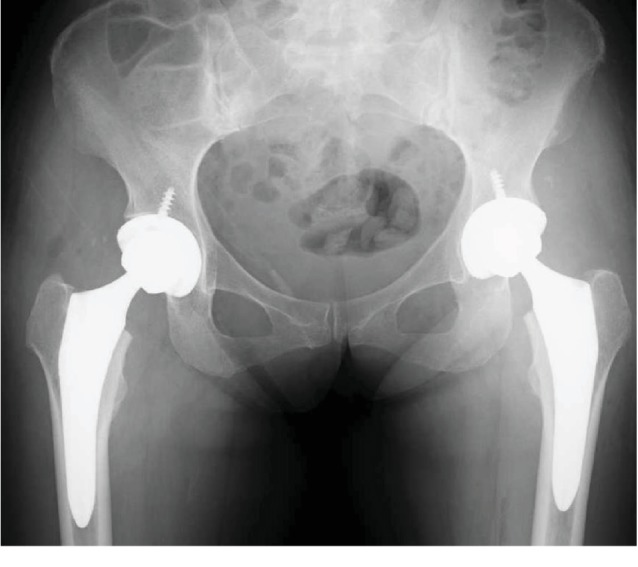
Postoperative radiograph of the bilateral THA.
